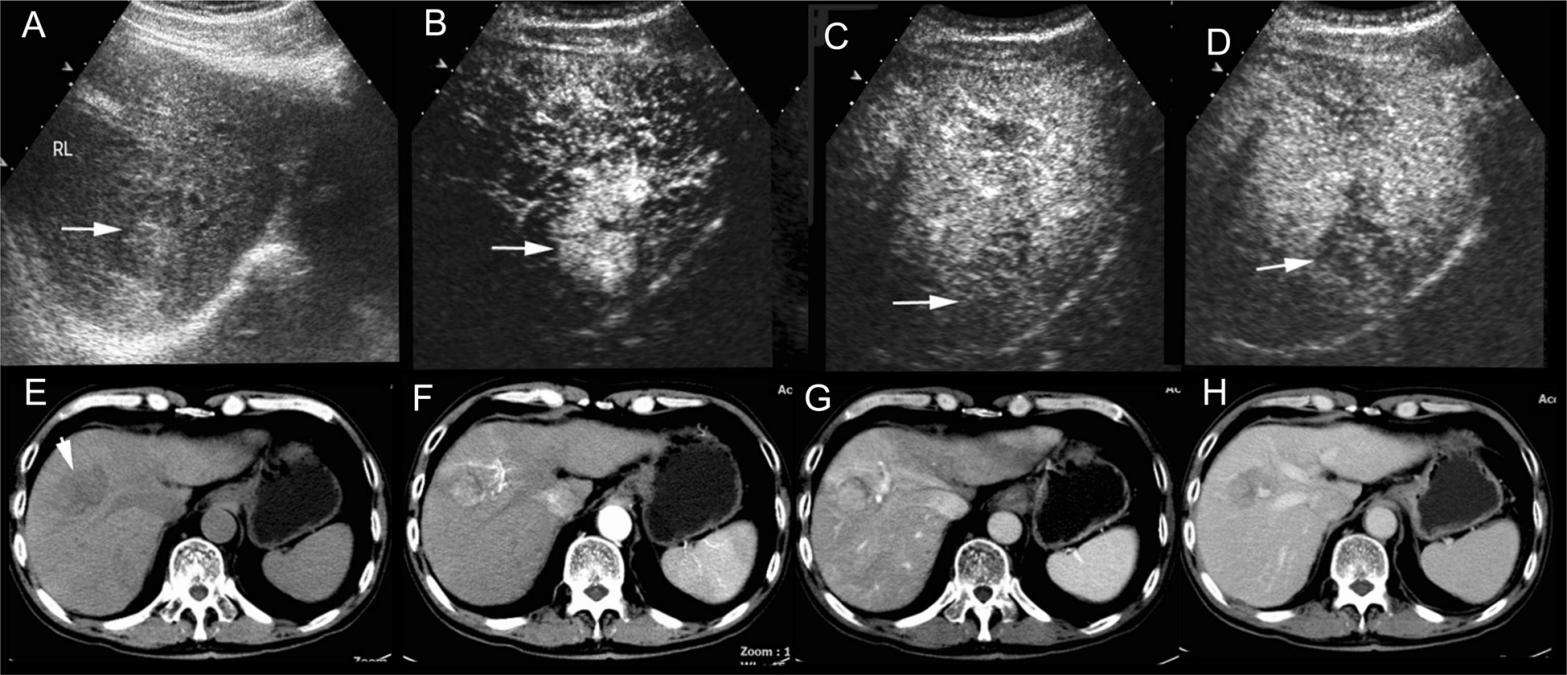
cHCC-CC in a patient with cirrhosis related to chronic hepatitis B infection. AFP test was normal and CA19-9 elevated (50.3U/ml) in discordance with presumptive imaging findings in the patient. Unenhanced ultrasound shows a hyperchoic mass of 3.7 cm with hypoechoic halo (arrow) in right lobe of the liver (a). The mass displays heterogeneous hyperenhancemen in the arterial phase (b, 29 s after contrast agent injection) followed by slow washout in the portal phase (c, 110 s after injection, arrow) and mild washout (d, 179 s after injection, arrow) in the late phase on CEUS, resembling the enhancement pattern of HCC. Unenhanced CT scan reveals a hypodense mass in right lobe of the liver (e, arrow). The mass displays heterogeneous hyperenhancement in the arterial phase (f) and the portal phase (g) followed by washout in the late phase (h) on contrast-enhanced CT, resembling the enhancement pattern of HCC
